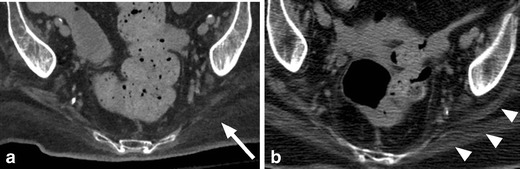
Marked muscular atrophy in patient 7 years after SCI. a Non-contrast CT image shows marked atrophy of the gluteal musculature with fatty replacement (*white arrow*). b The same patient, 7 years earlier, demonstrating baseline muscle mass (*white arrowheads*)
